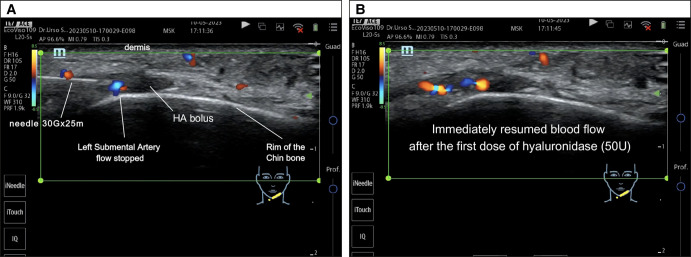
(A) Ultrasound examination showing a hyaluronic acid bolus blocking the left submental artery flow. Injection of hyaluronidase 50 IU, ultrasound-guided, directly into the bolus. (B) Ultrasound examination showing the immediate flow resumption following hyaluronidase injection.
